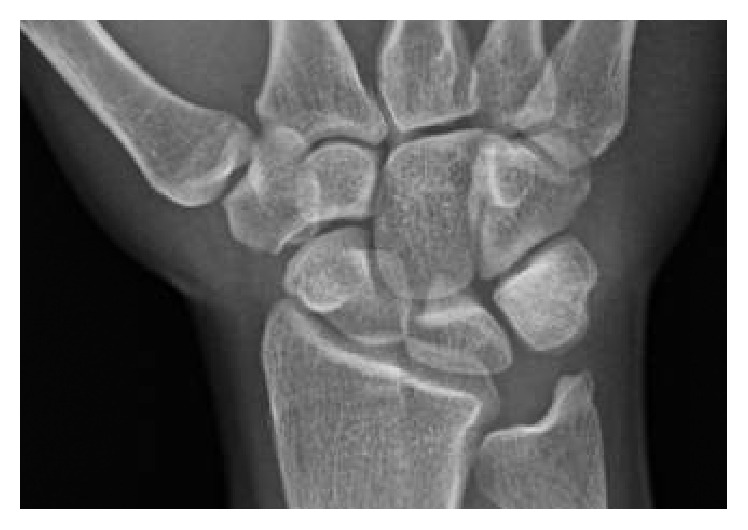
Posteroanterior radiographic view of the wrist at the time of initial evaluation that shows no abnormality in the scaphoid.
